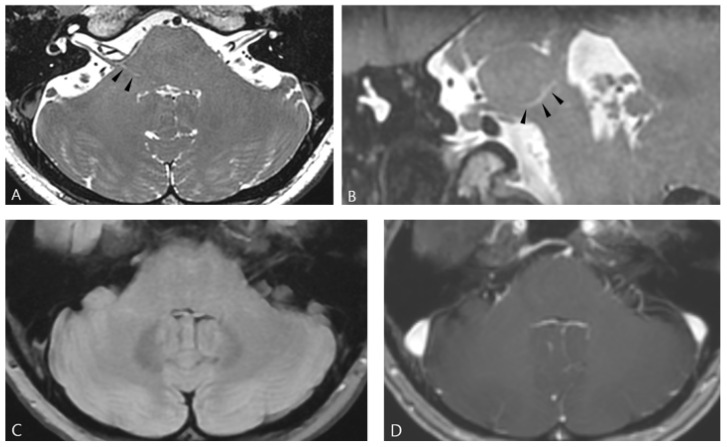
Axial (A) and oblique coronal (B) high-resolution proton density images (HR-PDI) demonstrate a curvilinear high-signal-intensity lesion along the presumed course of the intraparenchymal facial nerve fascicles in the right lateral pons (arrowheads). Conventional MRI sequences, including axial fluid-attenuated inversion recovery (FLAIR) (C) and post-contrast 3D T1-weighted imaging (D), show no definite focal pontine lesion.
